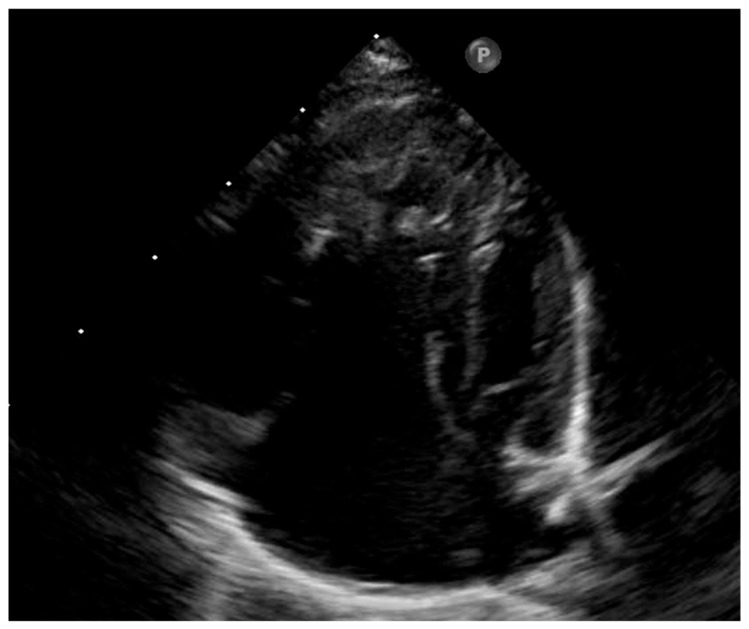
Neonatal echocardiographic four-chamber view showing marked dilation of the right-sided chambers, right ventricular dilation with hypertrophy, and hypoplastic left-sided cardiac cavities.
